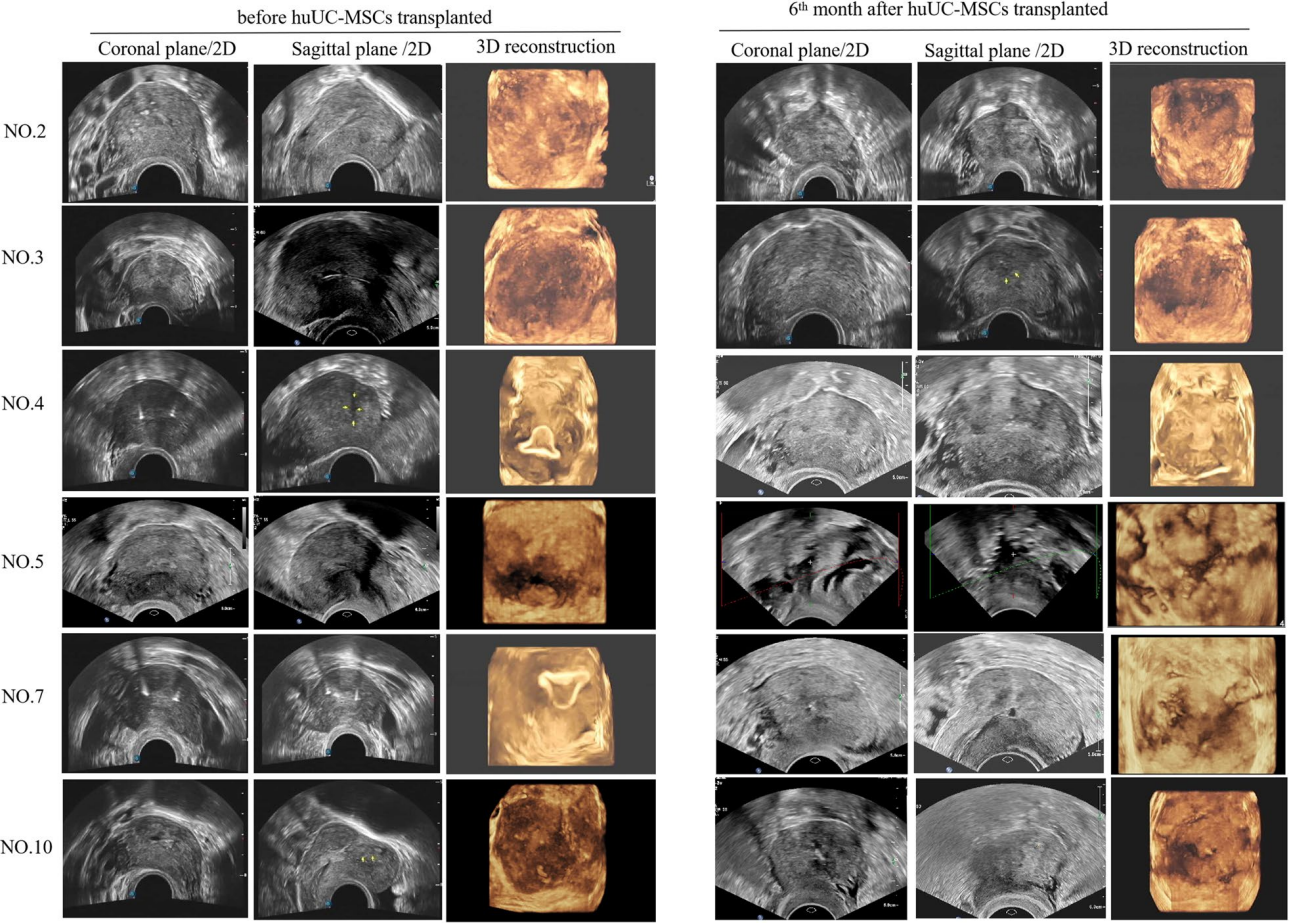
Doppler ultrasound imaging from 6 patients with intrauterine adhesion before and sixth month after human umbilical cord MSCs
